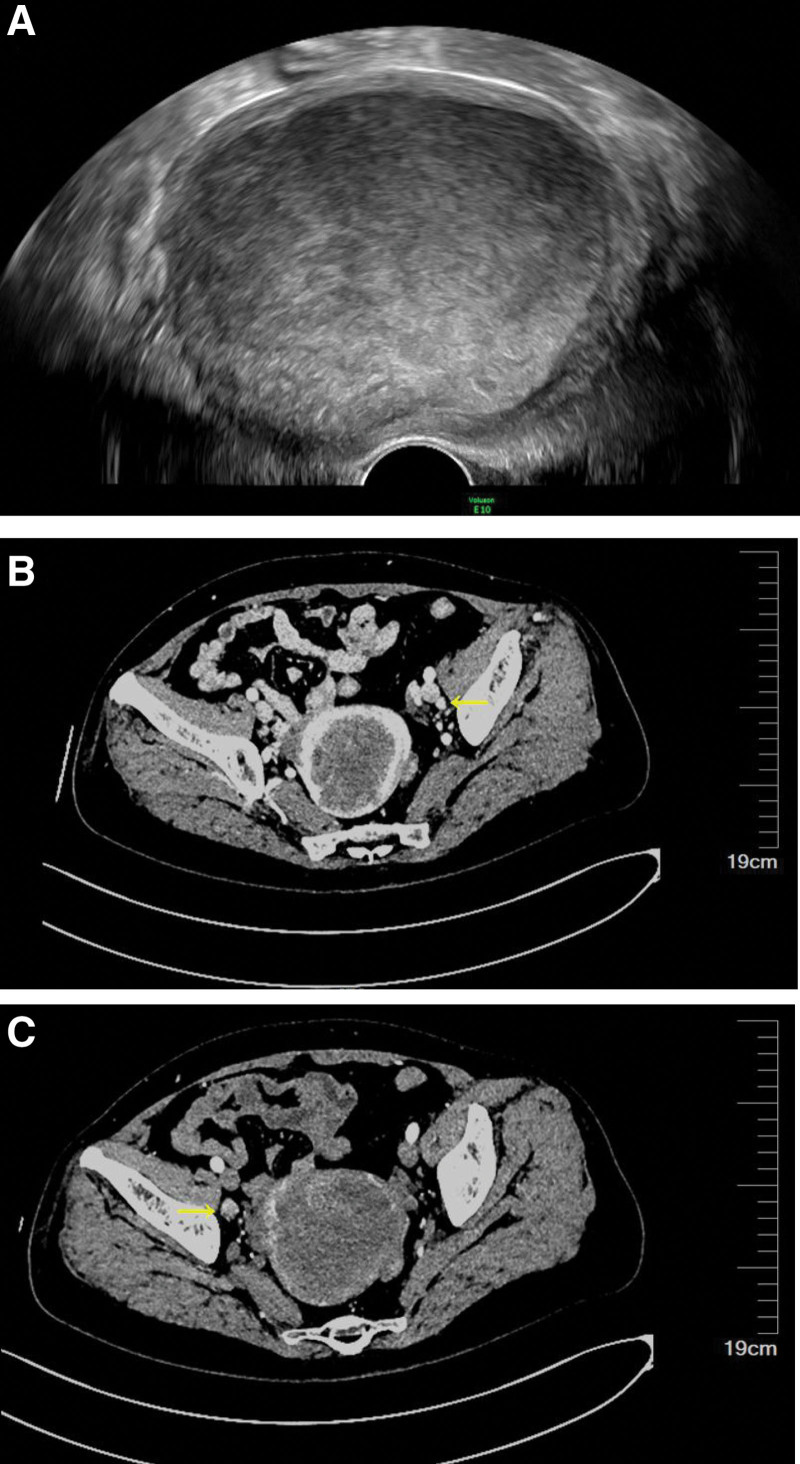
(A) Transvaginal ultrasonography revealed a hypointense, internally heterogeneous, space-occupying lesion measuring 90 × 77 × 73 mm in the uterine cavity. (B and C) Multiple lymph nodes beside bilateral iliac vessels in the pelvic cavity, some of which were swollen, were considered possible sites of metastasis.
